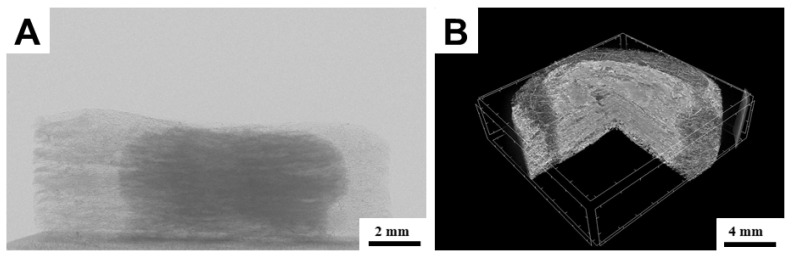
Computed tomography images visualizing the distribution of hydrogel within a scaffold structure. (A)-side view of the sample; X-ray scan. (B)-3D sample screening.
